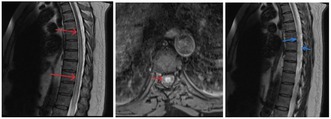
FIGURE 1 MRI shows medullary edema (red arrow) from T7 to L1 with the presence of perimedullary vessels (blue arrow).
